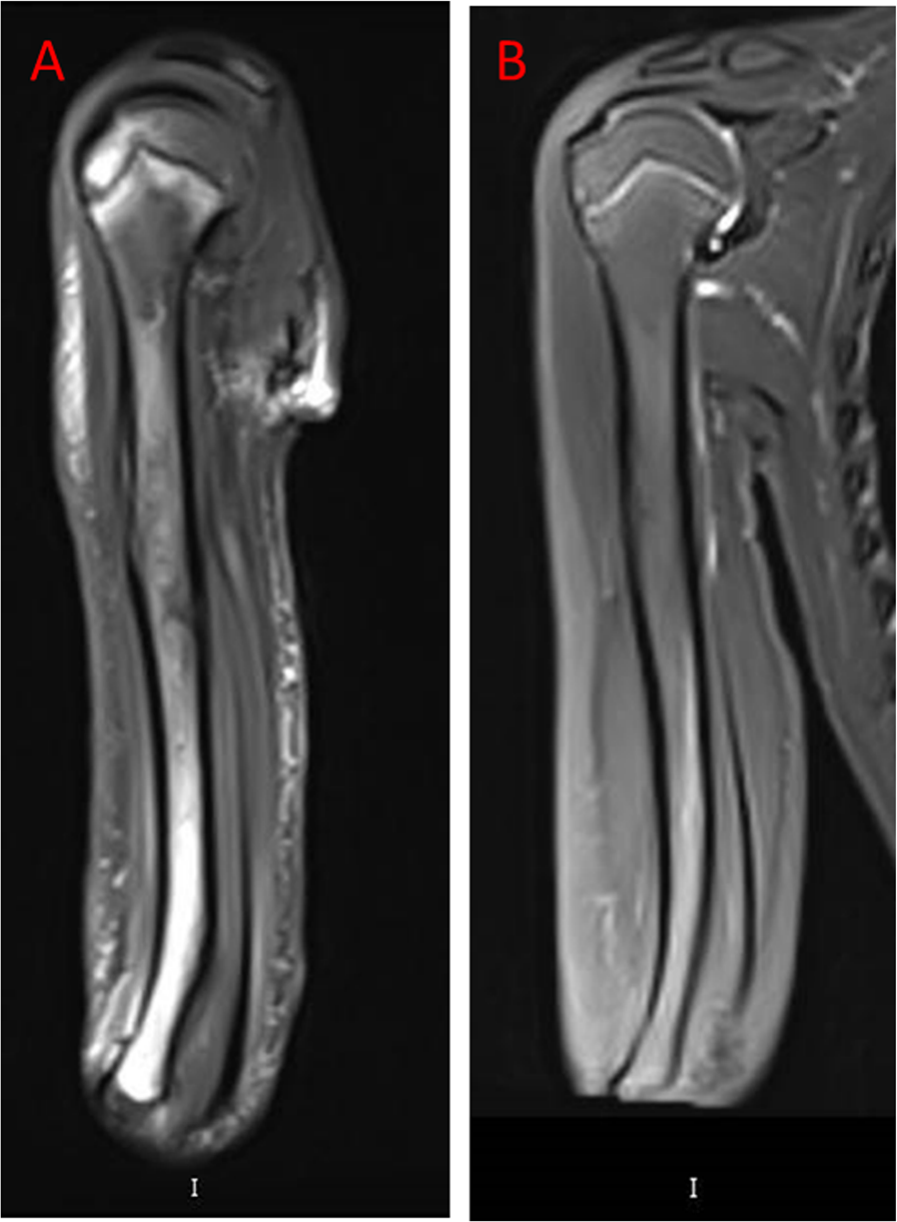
The MRI results of the right arm at first (a). At the follow-up MRI, the previous abnormal signals narrowed significantly (b)
